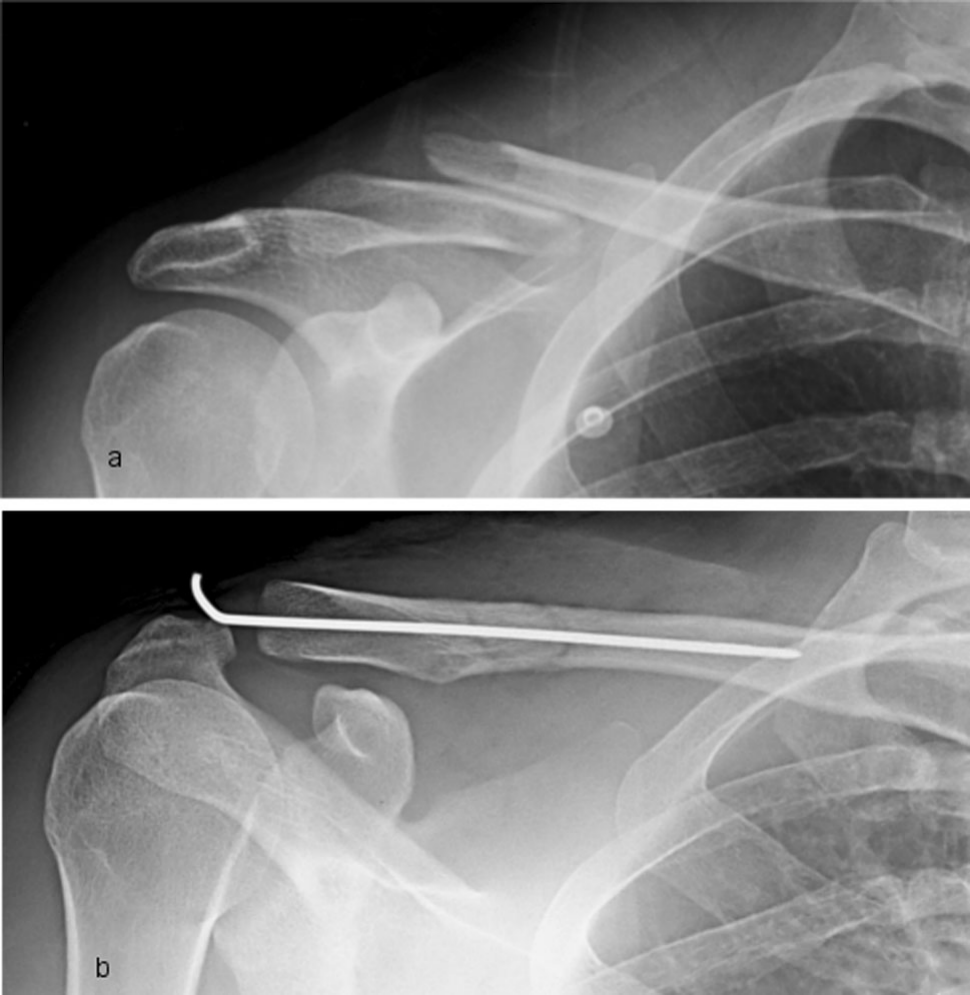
Figure shows the radiography of midshaft clavicle fracture a before and b after the surgical treatment
